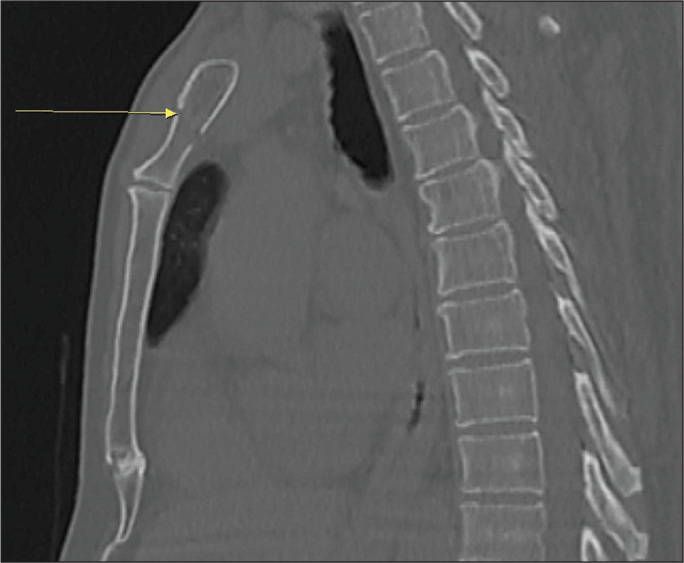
CT scan of a patient who suffered a fall from height, showing a linear
